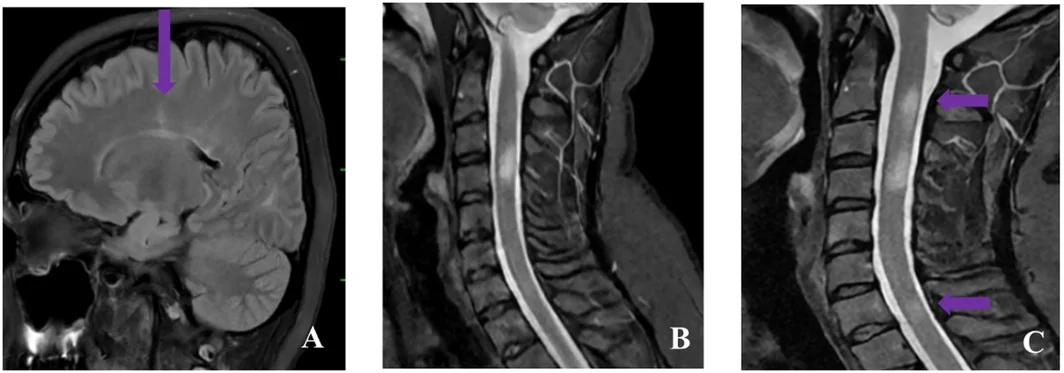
Brain MRI with typical MS lesions (A) and multiple periventricular lesions (Dawson's fingers sign) marked with an arrow (B). Short segment lesion in cervical MRI (C) and two new short segment lesions in the cervical cord after 5 months marked with arrows.
